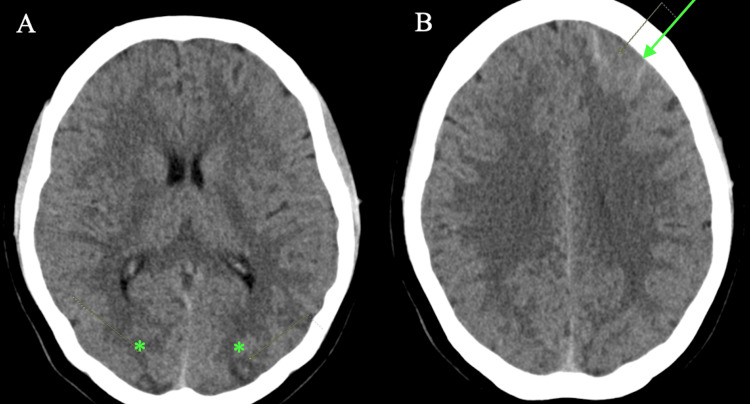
Noncontrast Brain CT (1A) Noncontrast CT indicating low-density areas in the cortical and subcortical region of both occipital lobes (*). (1B) Vague edema and minimal subarachnoid blood in the left frontal lobe (arrowhead)
